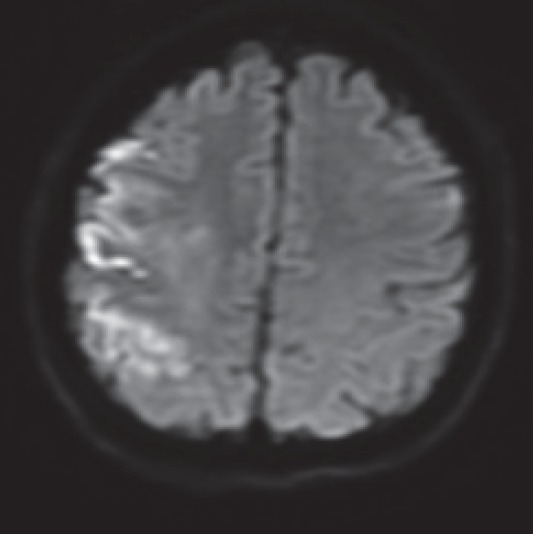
MRI diffusion sequence with right cortical fronto-parietal hyperintensity (the already known ischemic lesions), but also small left frontal new lesions
